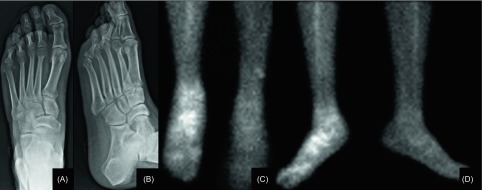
(A) Anteroposterior and (B) oblique right foot’s X-ray of 28-year old male, 15 days after he underwent a total hip replacement due to an advanced Legg-Perthes disease on his right hip, showing minimal osteoporotic changes on the right foot and ankle, especially at the tarsus and metatarsophalangeal joints. (C) Third-phase anteroposterior and (D) lateral bone scan images of both legs and feet of the same patient, displaying increased isotope accumulation along the right foot.
